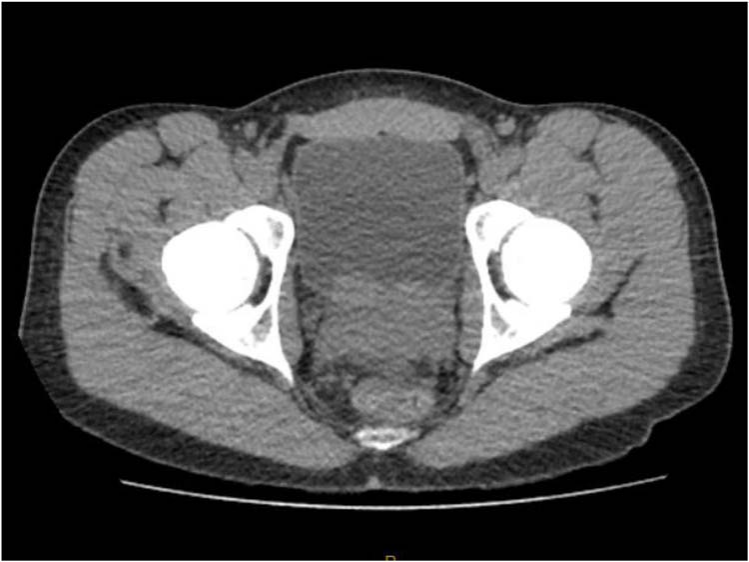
CT KUB showing irregularly enlarged prostate.
